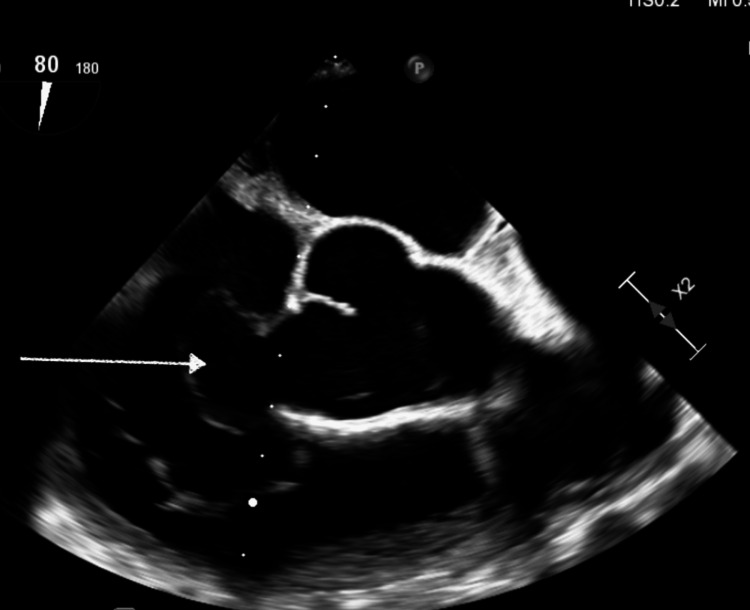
TEE image showing right SVA (arrow) TEE: Transesophageal echocardiogram, SVA: Sinus of Valsalva aneurysm
